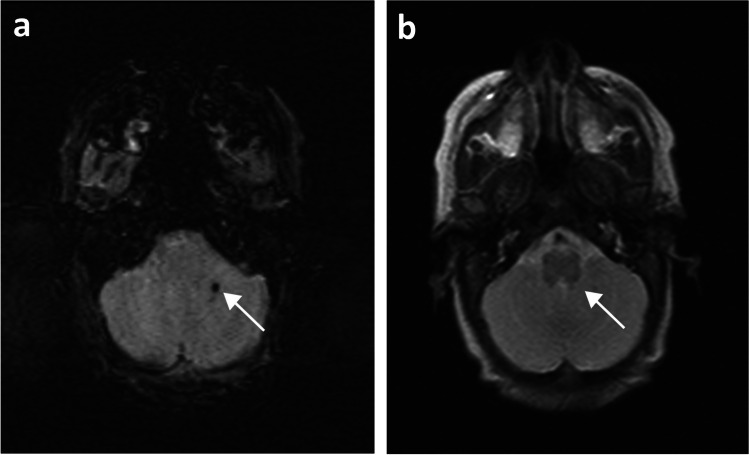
A 17-week-old girl born extremely premature at 23 gestational weeks with magnetic resonance imaging performed at term-equivalent age. a Axial susceptibility-weighted image shows a small cerebellar haemorrhage in the left hemisphere (*arrow*) with susceptibility-related signal loss. b Axial T2-weighted image shows cerebellar haemorrhage in the left hemisphere as a small hypointensity (*arrow*)
